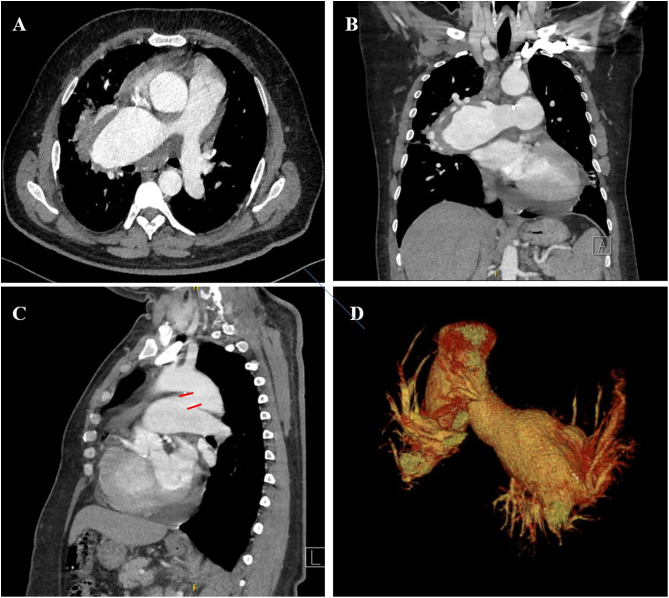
Contrast-enhanced chest CT scan shows an aneurysm of the right pulmonary artery in axial (A) and coronal (B) plane. Oblique plane (C) shows a patent ductus arteriosus marked with red lines. Image D represents a 3D reconstruction of the aneurysmal lesion.
